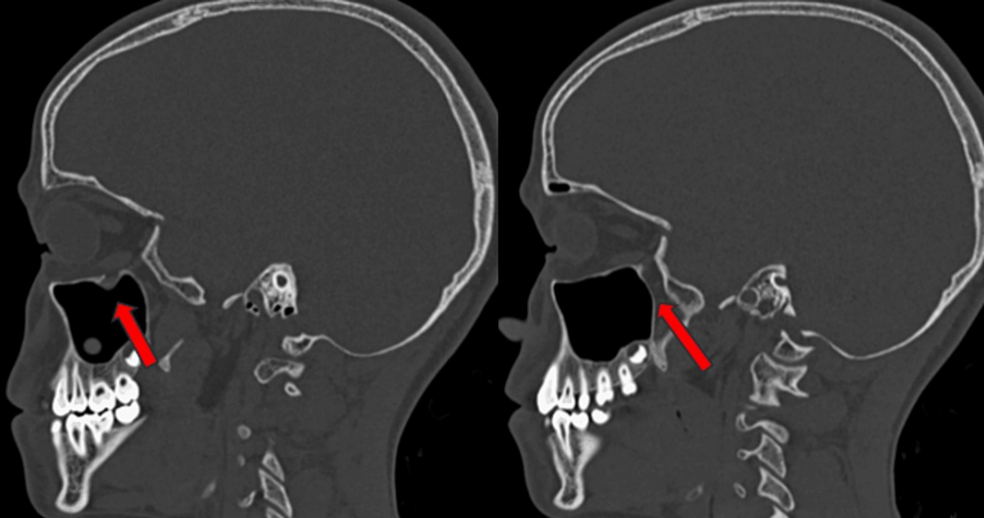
Case two: the sagittal section of NCCT face shows the release of entrapped muscles and orbital content and swing back to its position NCCT - non-contrast computed tomography
